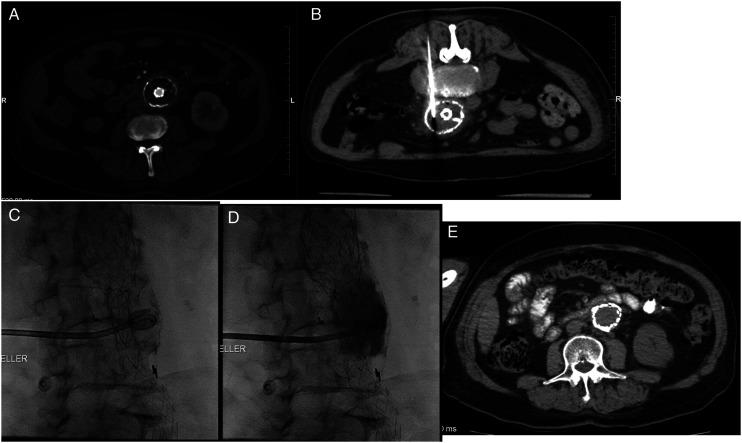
A: Prior infrarenal aorto uni-iliac stent graft. Gas-containing, fluid filled aneurysm sac which had demonstrated progression in size compared to previous (not depicted). B-D: CT- and fluoroscopy-guided placement of 10Fr drain into aortic sac via left posterior approach. E: Drain was removed 59 days following placement. CT at approximately 2 years followup demonstrates no evidence of residual collection or peri-aortic inflammatory change.
